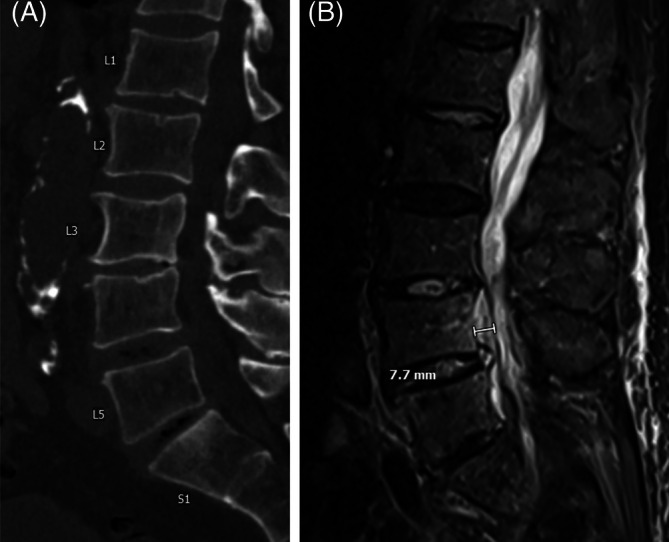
(A) Sagittal computed tomography of the lumbar spine without contrast demonstrating no visible epidural collection. (B) Sagittal magnetic resonance imaging of the lumbar spine performed 1 week later revealing a 7.7‐mm epidural abscess over L4–L5 vertebral bodies.
